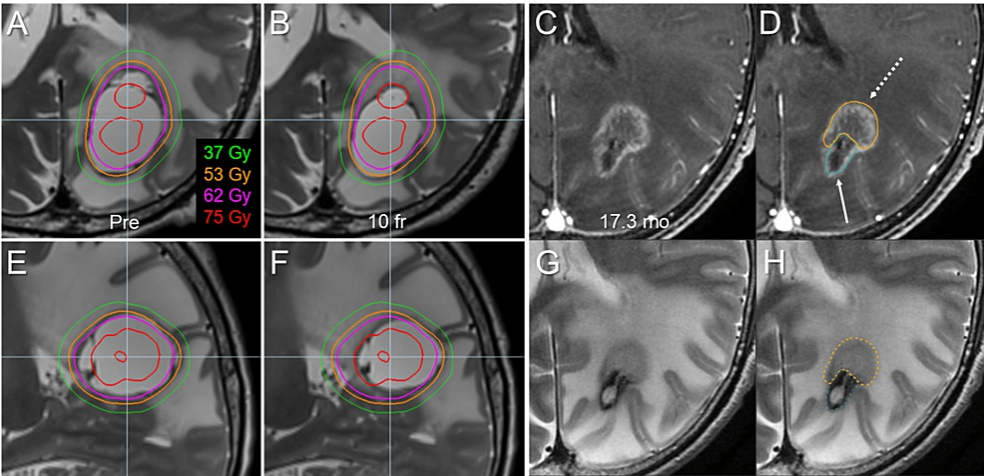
Discrepancy between planned and actually absorbed doses at the completion of stereotactic radiosurgery and its comparison with magnetic resonance images before lesionectomy. Axial images (A-D, G, H); sagittal images (E, F); T2-WI (A, B, E-H); CE-T1-WI (C, D); before fSRS (pre) (A, E); at 10 fr (B, F); at 17.3 months after fSRS, just before craniotomy (C, D, G, H). Planned representative isodose lines are superimposed onto T2-WI (A, B, E, F). Of note, the maximum dose to the adjacent brain parenchyma finally increased up to 7.5 Gy per fraction at the ventral side of the GTV (B, F). On T2-WI, the dorsal low-intensity stable remnant (highlighted in light blue, arrow in D) and the ventral iso-intensity blurry-demarcated enlarging component (orange, dashed arrow in D) are contrasting. CE: contrast-enhanced; T1-WI: T1-weighted image; T2-WI: T2-weighted image
